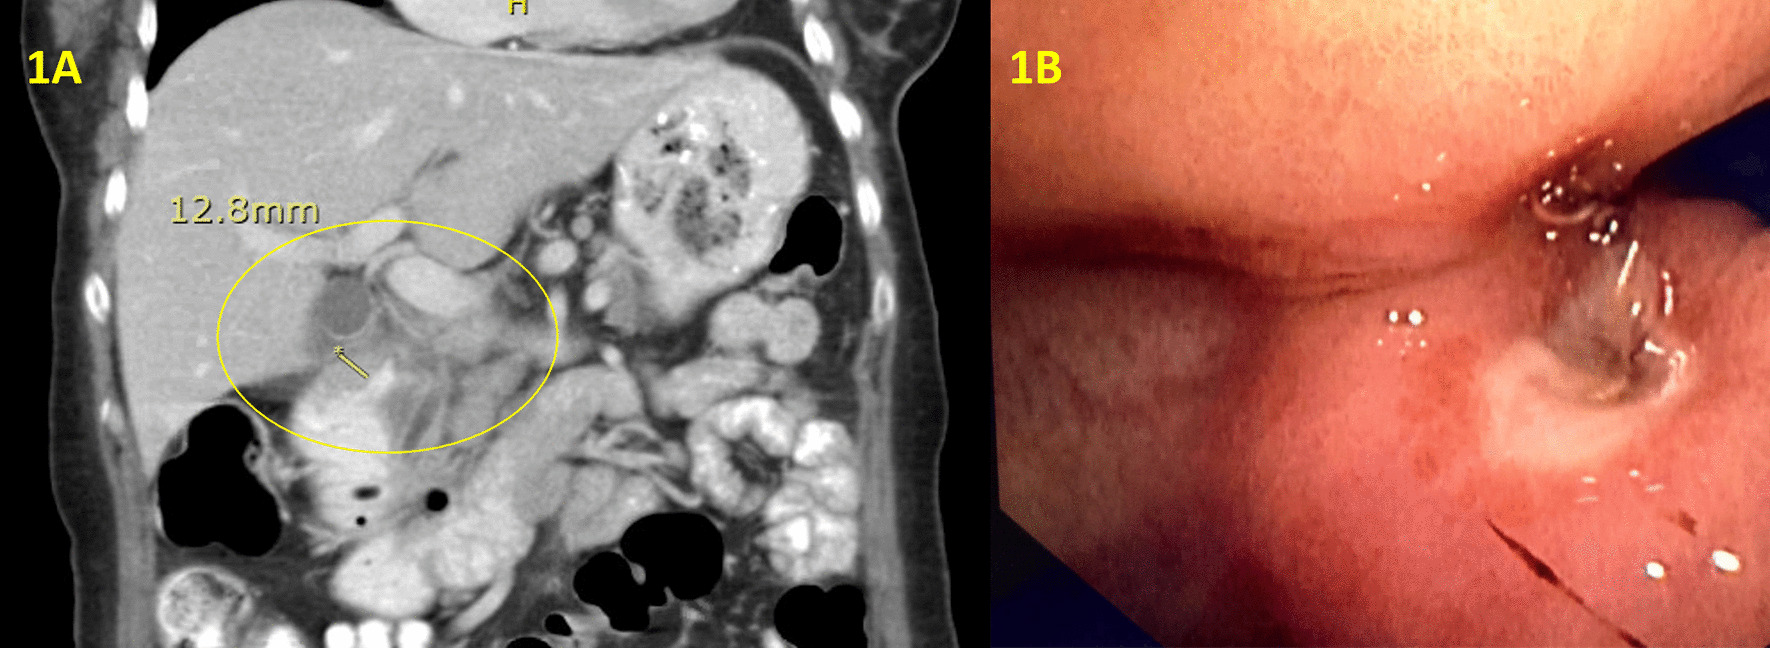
Preoperative CT showed mural thickening of the proximal duodenum (A). Gastroscopy showed a clean based antral ulcer extending into the duodenum with severe obstructing duodenal stricture (B)
